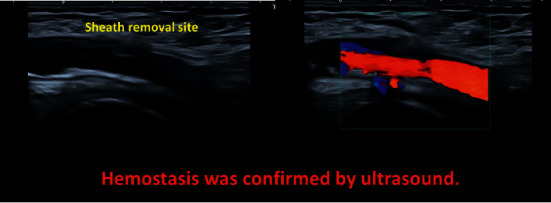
Confirmation of hemostasis by ultrasound.
